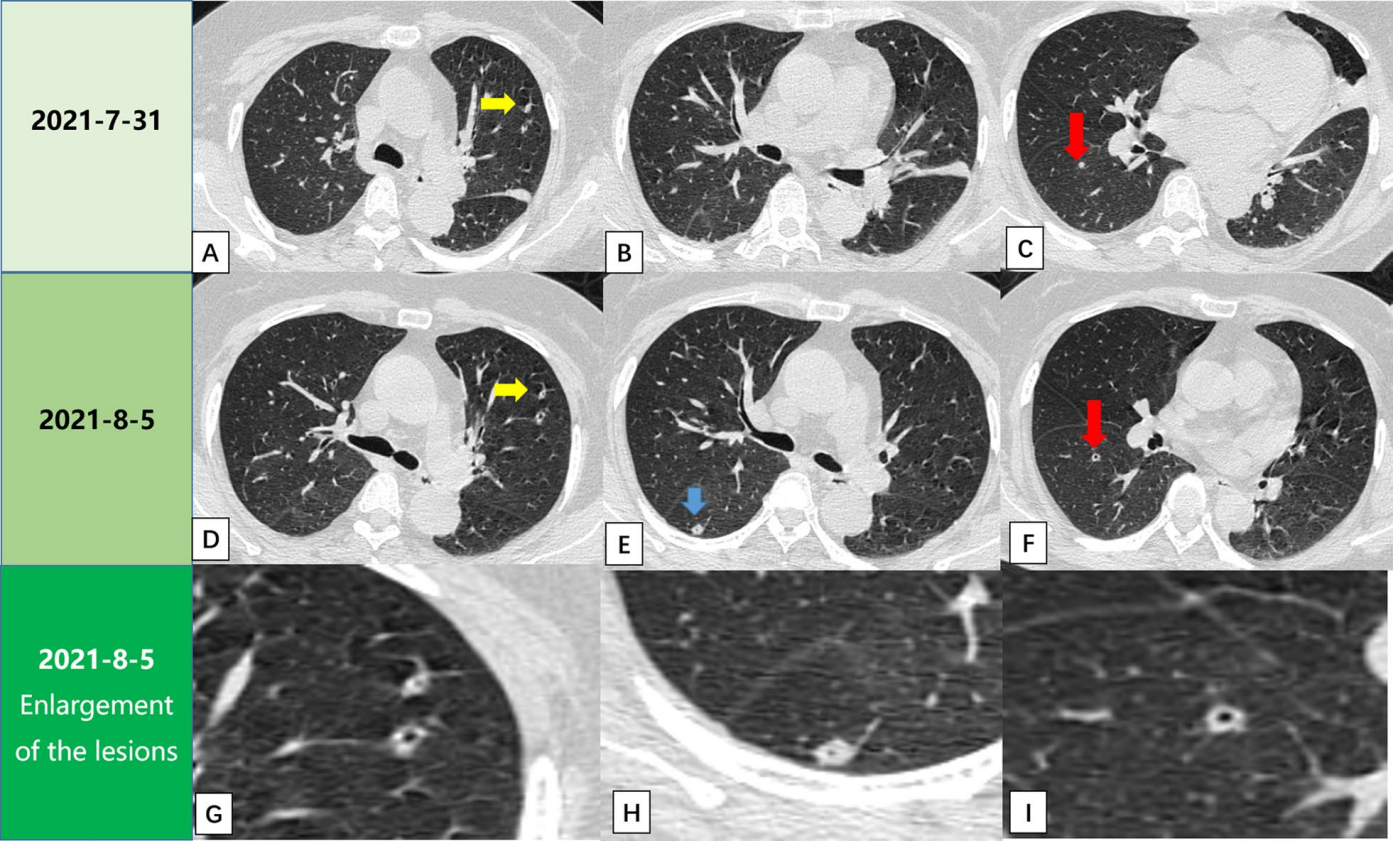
The comparison between the two CT examinations of the patient. A–C are the initial chest CT on July 31, 2021, showing patchy high-density shadows in the lingual segment of the left upper lobe, and multiple nodules in both lungs, as shown by the yellow arrow in A and the red arrow in C. D–F is a follow-up CT with the same slice as the previous images. Compared with A, D shows small cavity in the left pulmonary nodule, as shown in the enlarged G. Compared with B, E shows a new nodule in the right pulmonary lung as the blue arrow. The enlargement image in H shows a small cavity in the center. Compared with C, F shows the patchy high density has disappeared in the left lung and a small cavity appears in the nodule of the right lower lobe. The enlargement image in I shows a small cavity in the center
